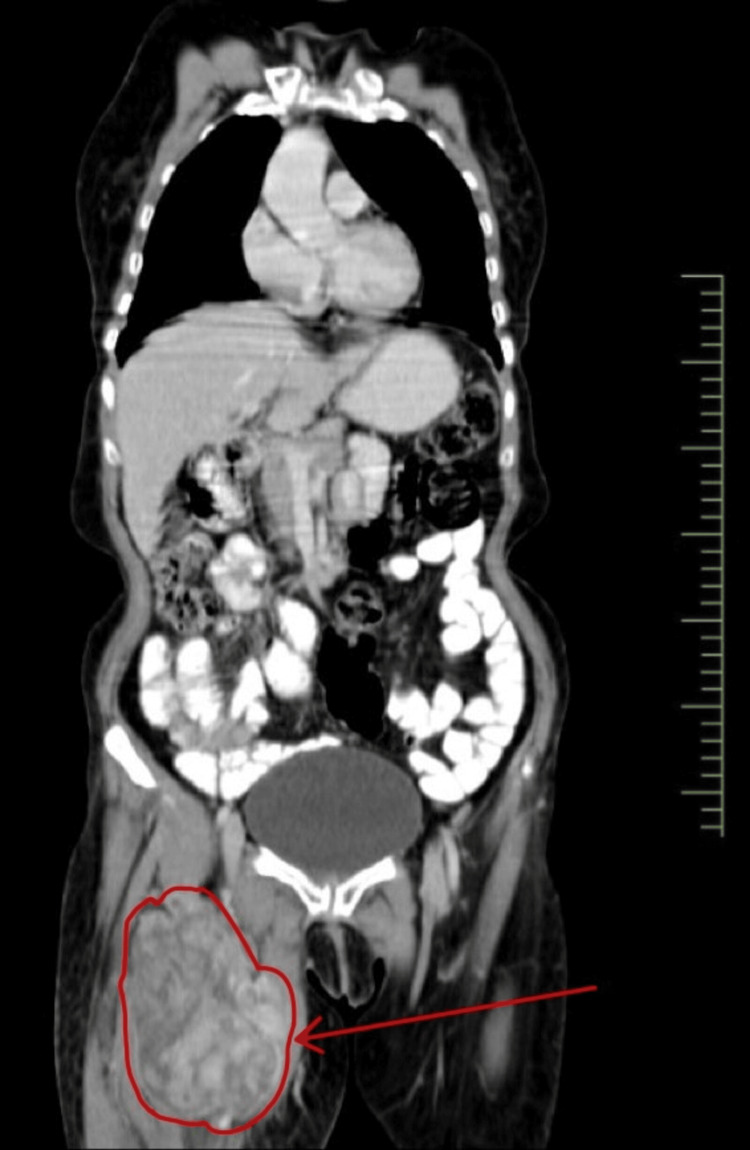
Contrast-enhanced CT showing an ill-defined heterogeneously enhancing lesion measuring 13 x 8.5 cm in the right proximal thigh.
